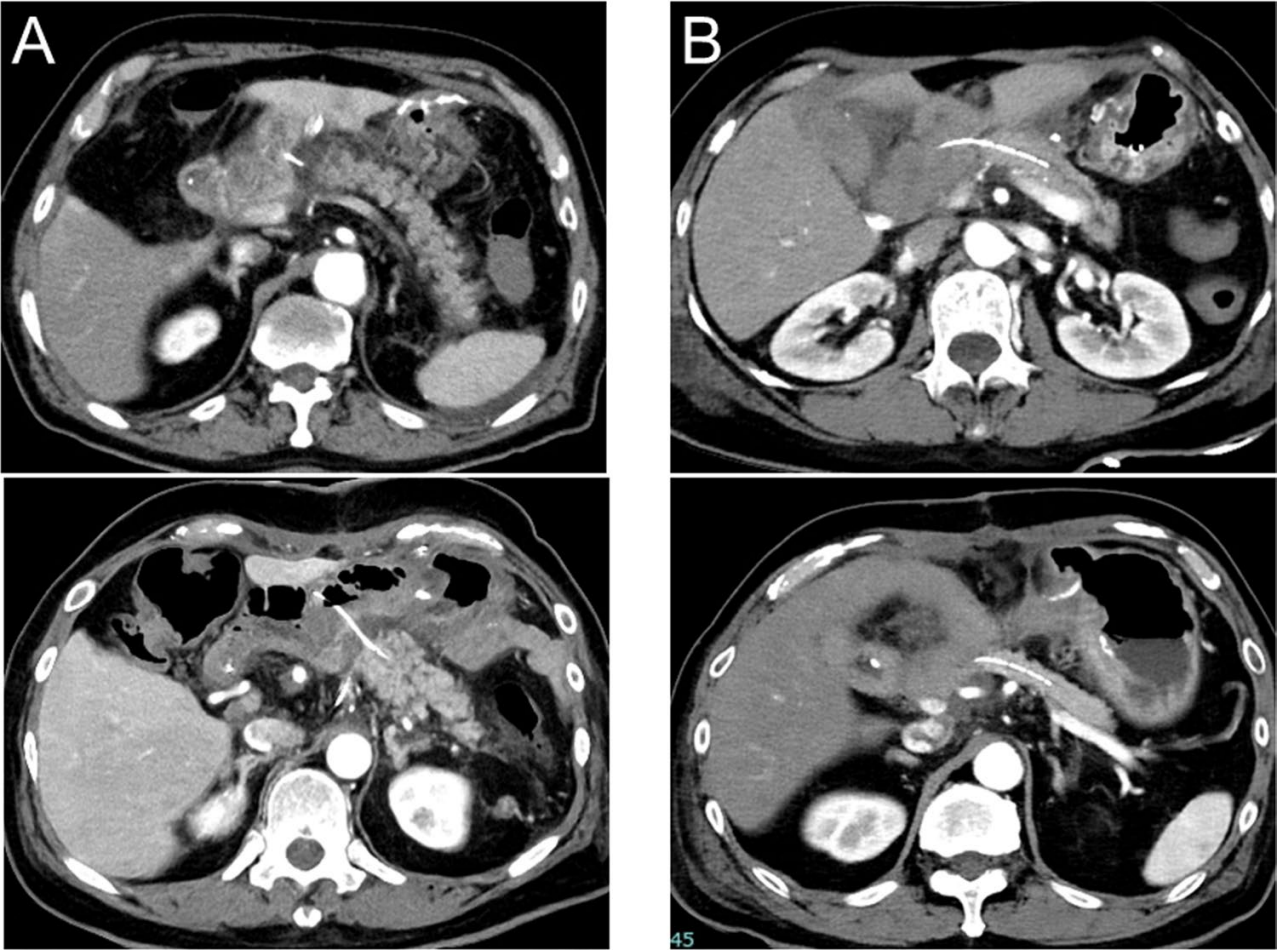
Representative images of E-HA and S-HA. The pictures show representative image of E-HA (A) and S-HA (B) on CE-CT. CE-CT, contrast-enhanced computed tomography; E-HA, evident hypoattenuated area; S-HA, subtle hypoattenuated area
